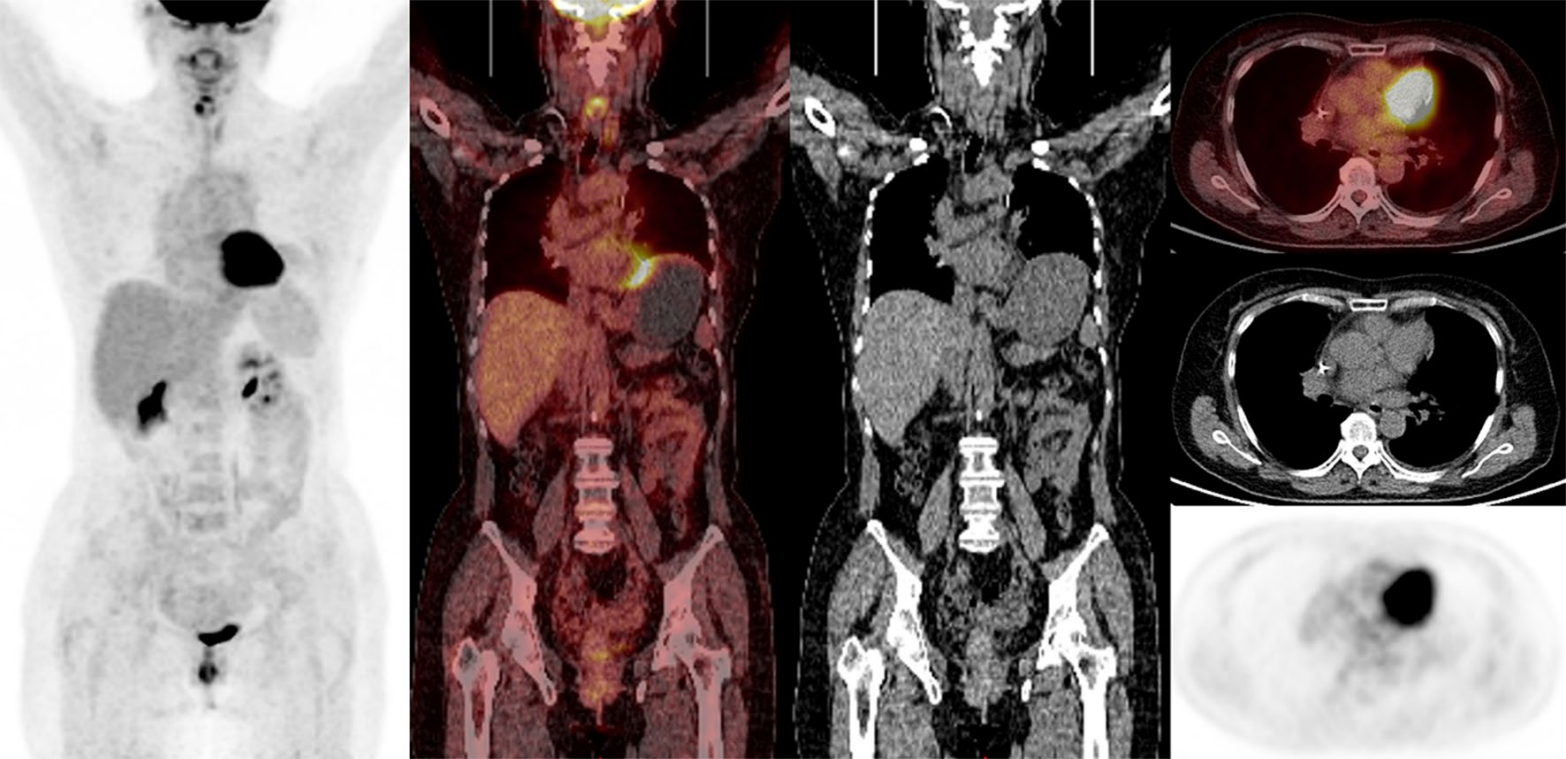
Representative images of an FDG PET/CT scan in a patient who was treated with surgery and adjuvant radiotherapy for stage III thymic carcinoma. Restaging PET/CT was negative. The patient remained disease-free during the follow-up period.
